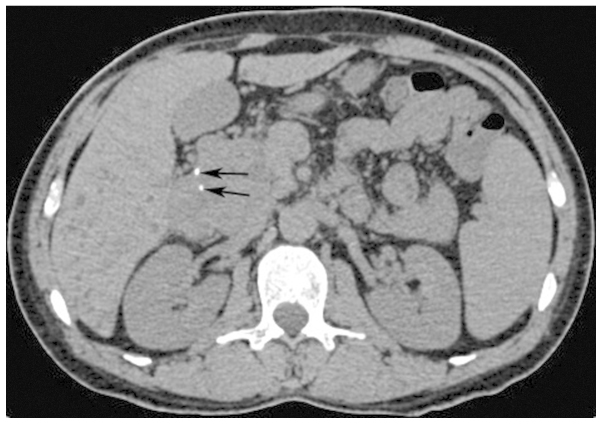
A 46-year-old male with a low-grade extragastrointestinal stromal tumor in the mesentery. Axial plain computed tomography image showing a 5.2-cm isodense mass with calcification (arrows).
